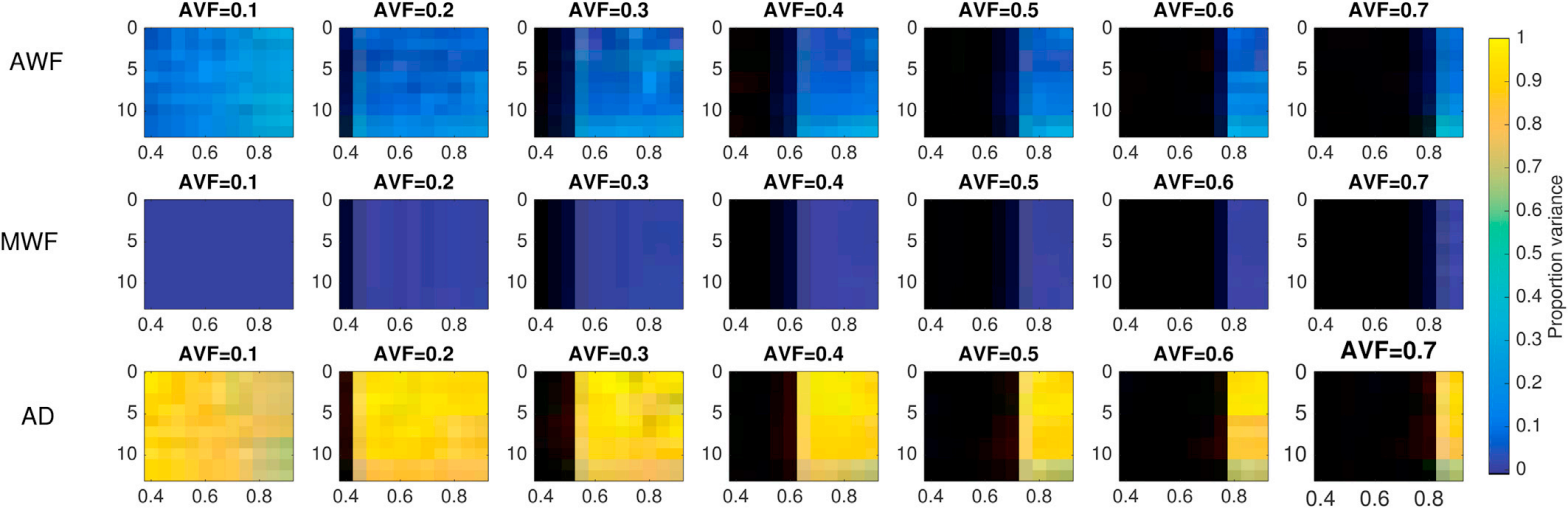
Proportional variance explained by noise in MRI acquisition, across the parameter space tested.
